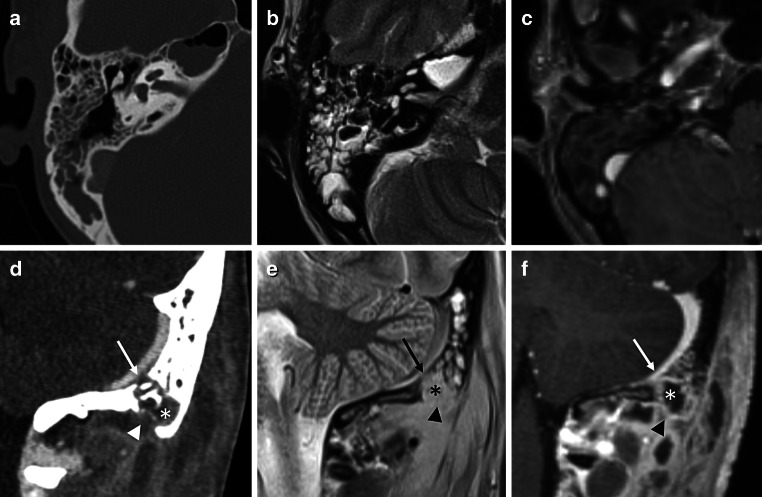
Images of the right temporal bone in CT (a), MRI, T2 TSE (b), and MRI, T1 Gd MPRAGE (c) showing no bone destruction. Images of the left temporal bone in  CT (d), MRI T2 TSE (e), and MRI T1 Gd MPRAGE (f) showing bone destruction of septa (*asterisk*), inner cortical table (*arrow*) and outer cortical table (*arrowhead*) of the mastoid
